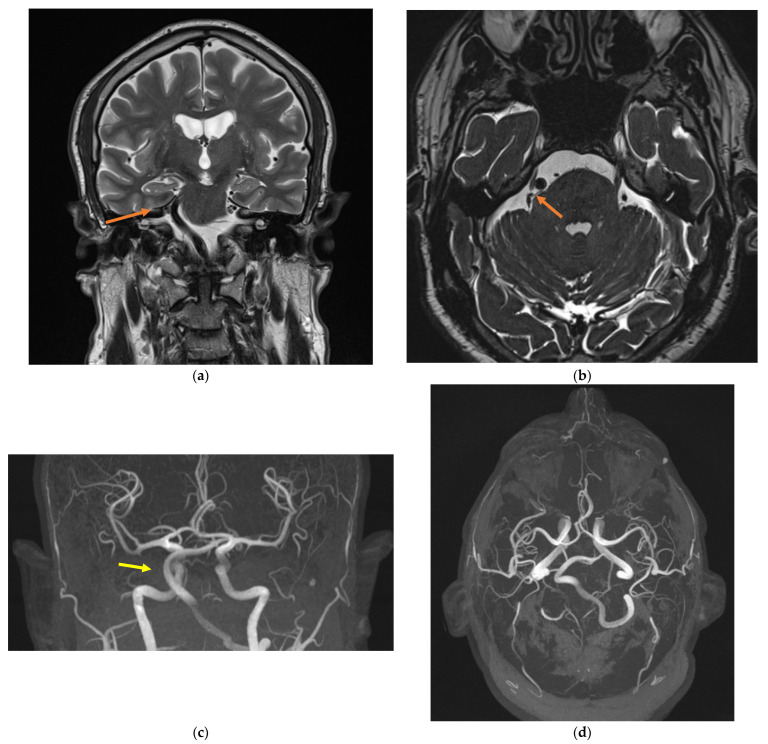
Magnetic resonance imaging showing the neurovascular conflict (a,b; orange arrow) and the vertebrobasilar dolichoectasia (c,d; yellow arrow). (a): coronal plane, T2-weighted sequence; (b): axial plane, 3D-CISS sequence; (c): coronal plane, MRI angiogram; (d): sagittal plan, MRI angiogram.
